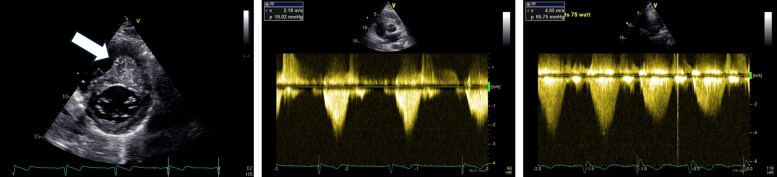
Continuous-wave Doppler signal in right ventricular outflow tract obstruction. Left panel shows a hypertrophic right supraventricular crest of the right ventricle (arrow) with an RVOT gradient of 19 mmHg at rest (central panel), increasing to an RVOT obstruction peak gradient of 66 mmHg with typical dynamic dagger-shaped CWD signal during bicycle stress echocardiography (right panel).
